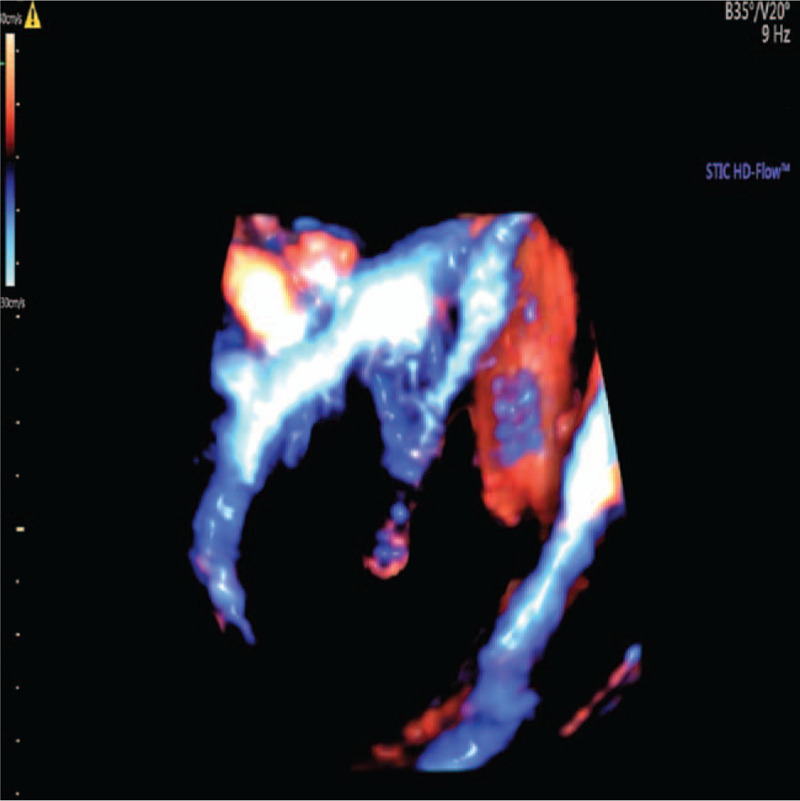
Ultrasonic features in power Doppler. The mass appeared to be highly vascularized around the lesion but with less vessels inside (dark part in center).
